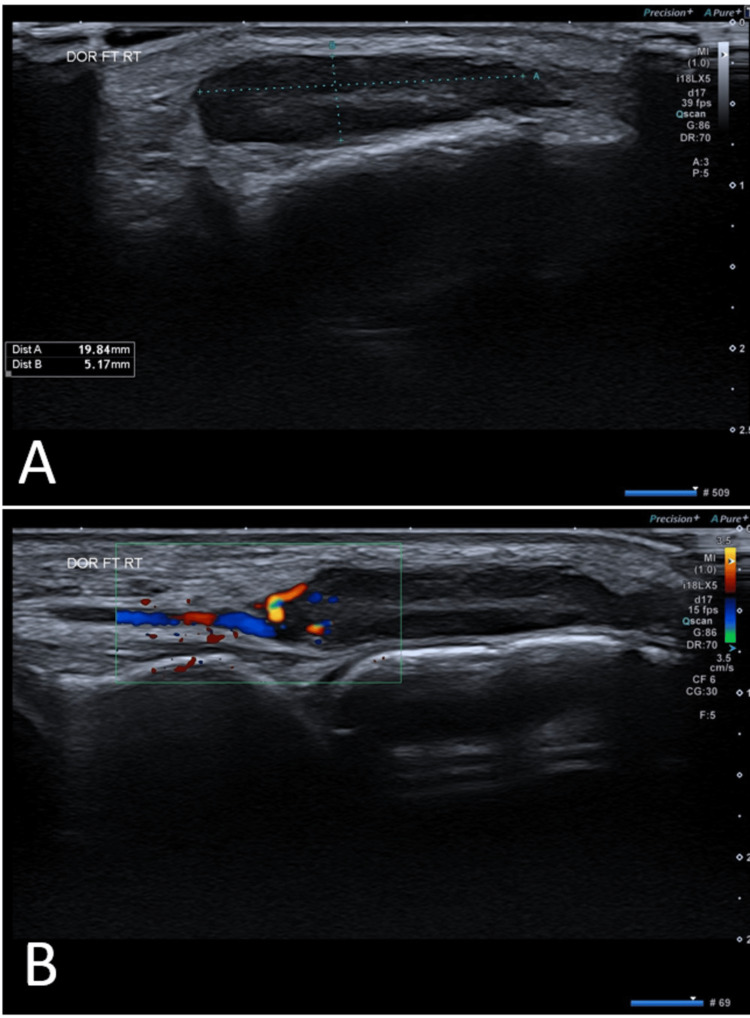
Ultrasound showing a heterogeneous well-circumscribed mass on the dorsum of the right foot measuring 19 x 5 x 4 mm
